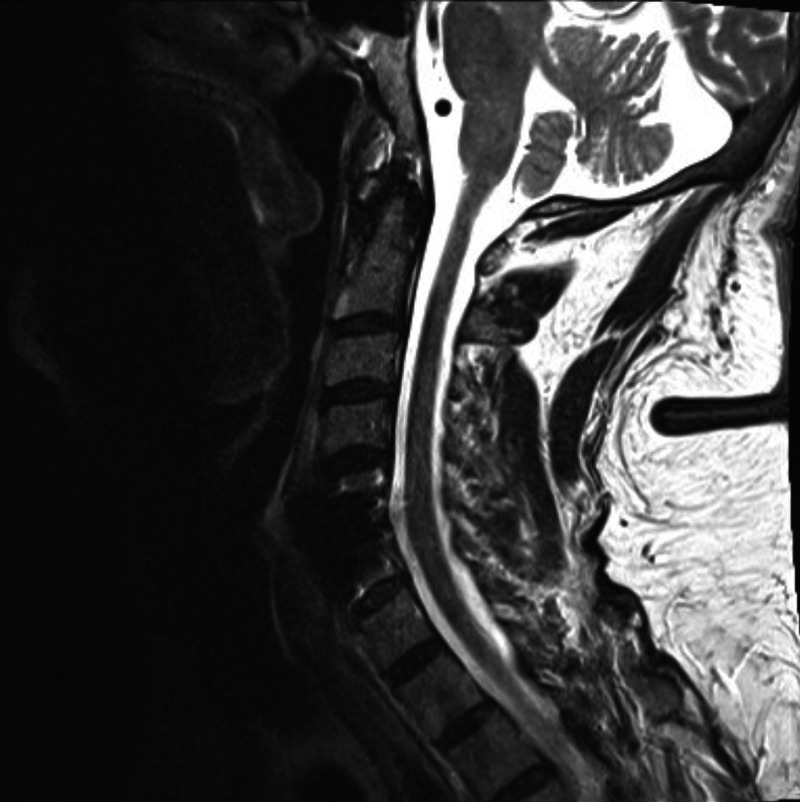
Cervical spine MRI obtained during admission
